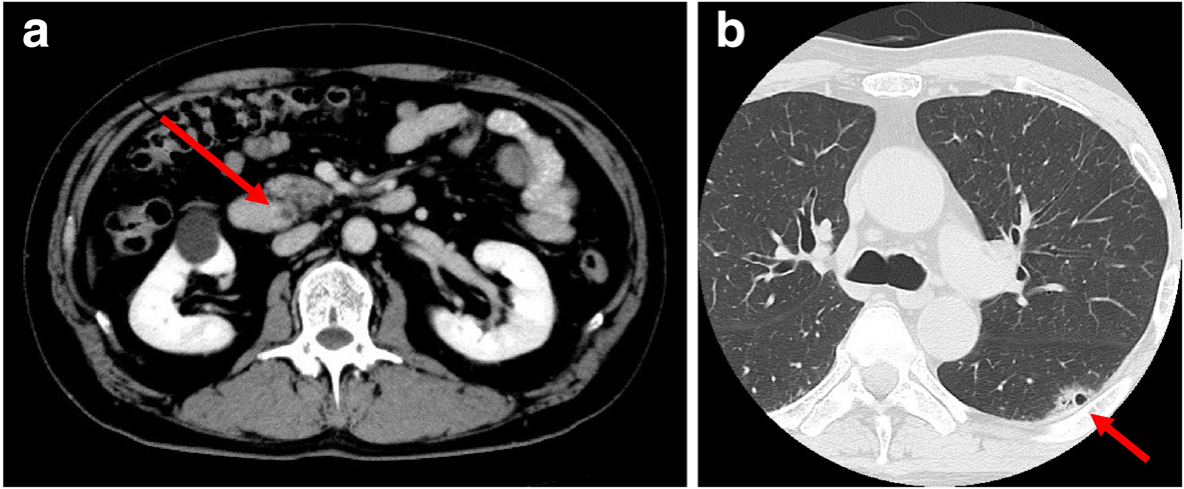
Computed tomography findings. a Abdominal computed tomography showing thickening of the distal bile duct (*arrow*). b Chest computed tomography showing an air-space consolidation in the upper lobe of left lung (*arrow*)
